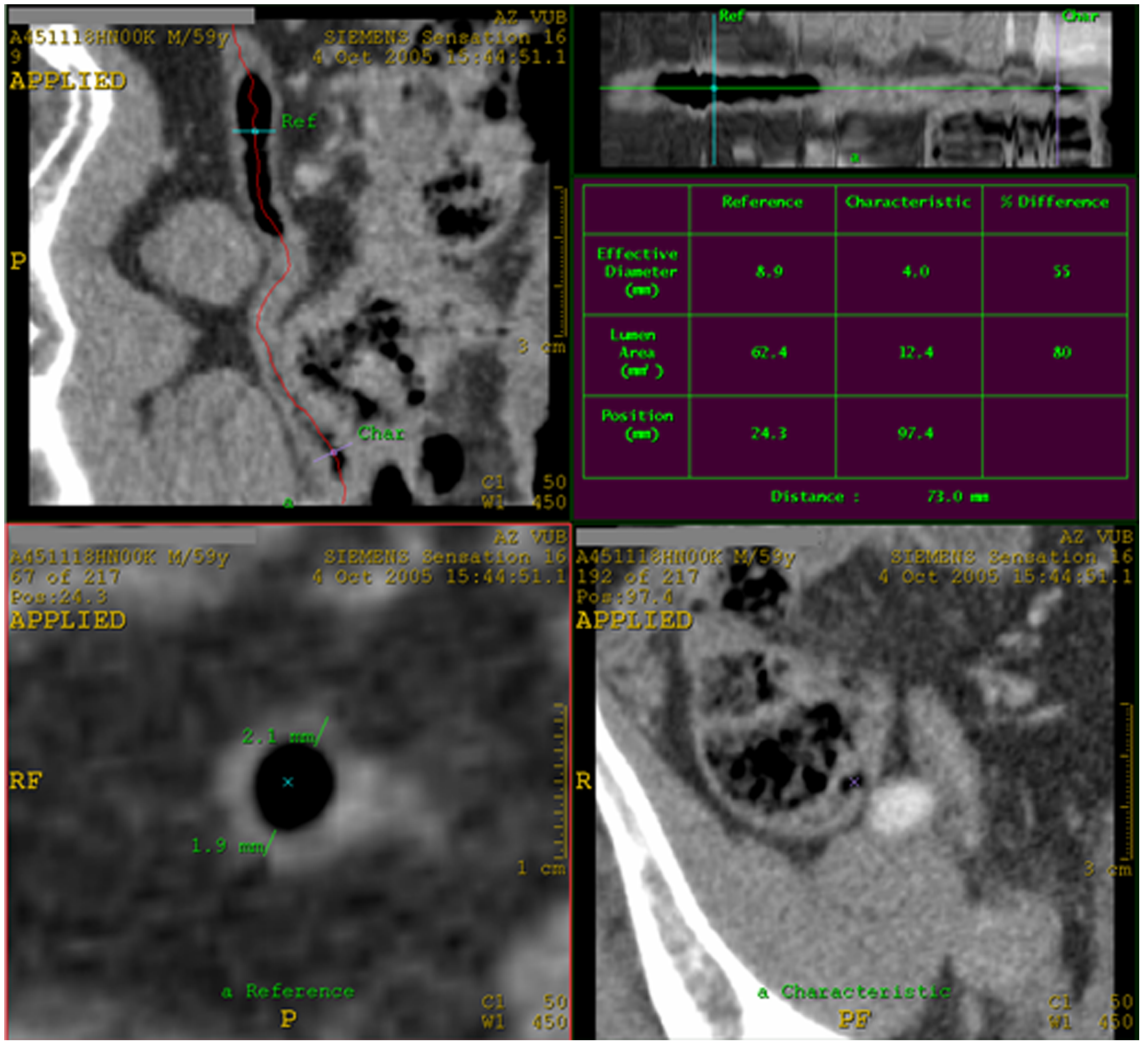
Measurement of the minimum and maximum wall thickness of the normal appendix on CT. In the top left side viewport the appendix was visualized along its complete length. Here the reader looked for the image truly perpendicular to the axis of the appendix, which corresponded to the wall thickness of the appendix. The wall thickness was measured in the two opposite walls on an axial image in the bottom left side viewport. In the top right side viewport, the appendix was seen as a linear structure.
